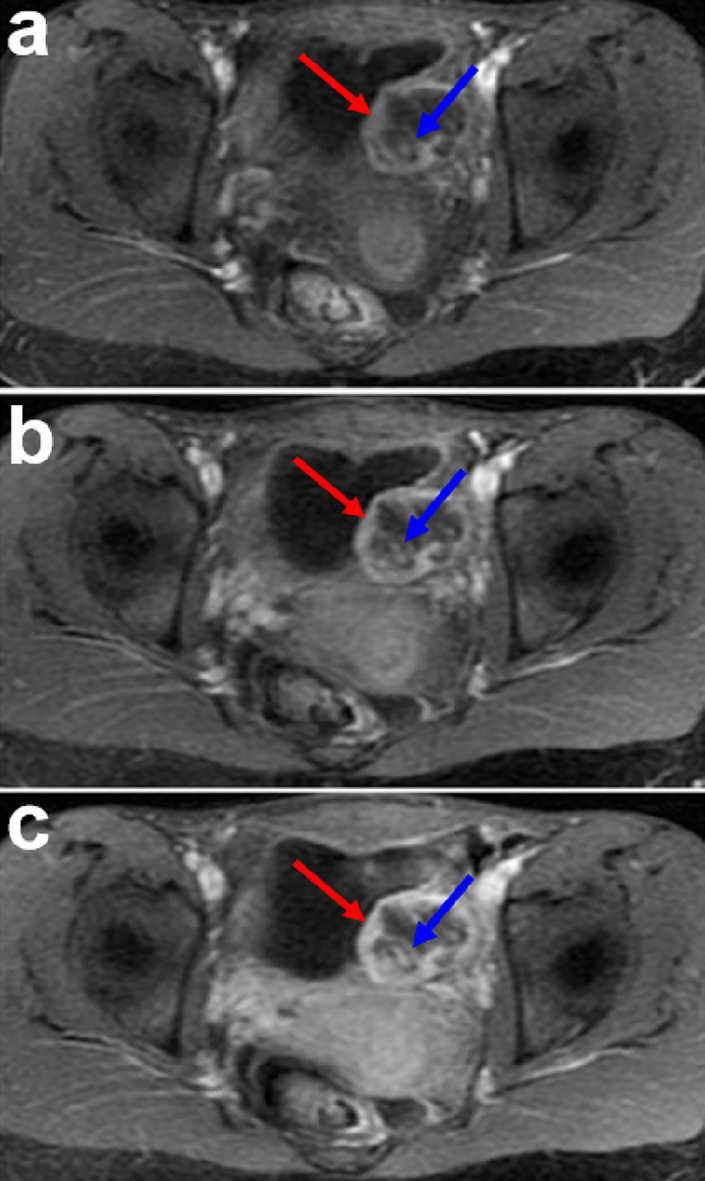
Contrast enhanced MRI of the IMT. (a-c) Axial gadolinium-enhanced fat-suppressed *T*
